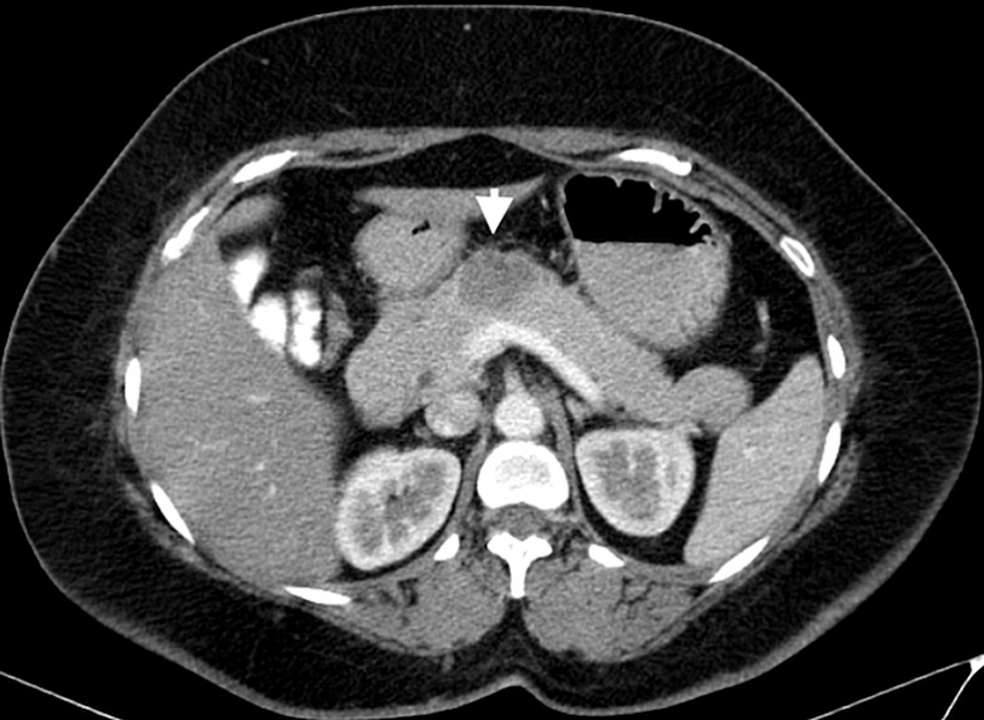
Contrast-enhanced CT abdomen image in portal venous phase showing a heterogeneously enhancing lesion in the neck and body of pancreas with normal main pancreatic duct and patent splenic vein.
